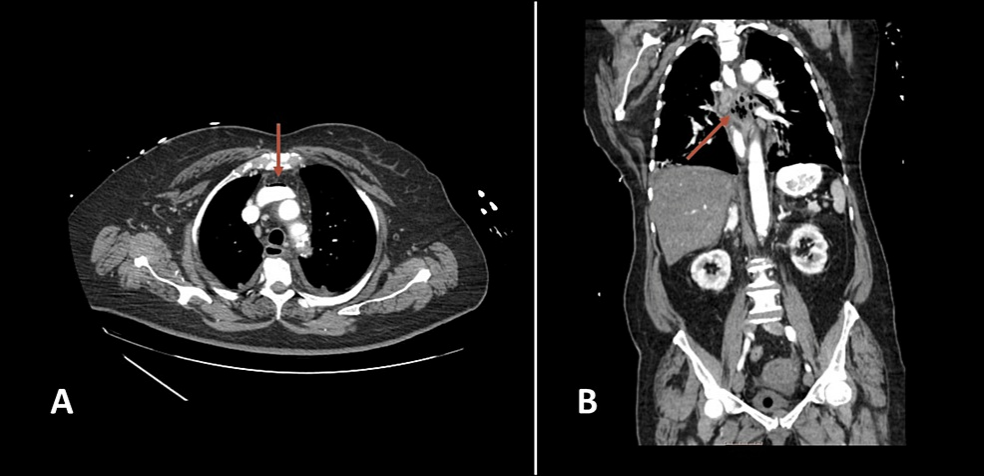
Contrast-enhanced thoracoabdominal CT Axial (A) and coronal (B) sections of contrast-enhanced thoracoabdominal CT demonstrating pneumomediastinum (arrows) CT: computed tomography
